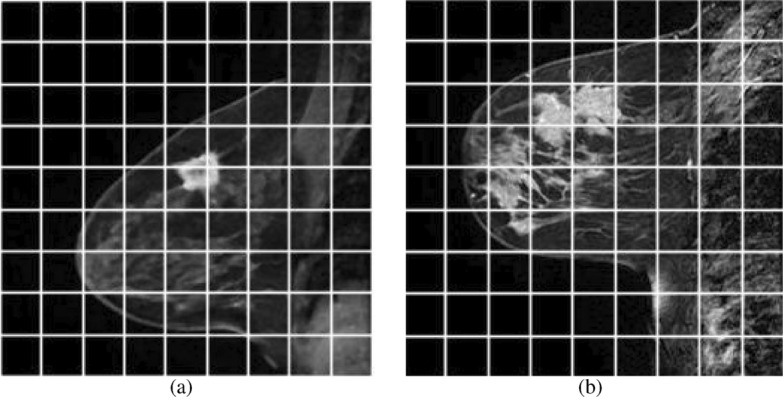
Original T2 weighted sagittal DCE-MRI.
